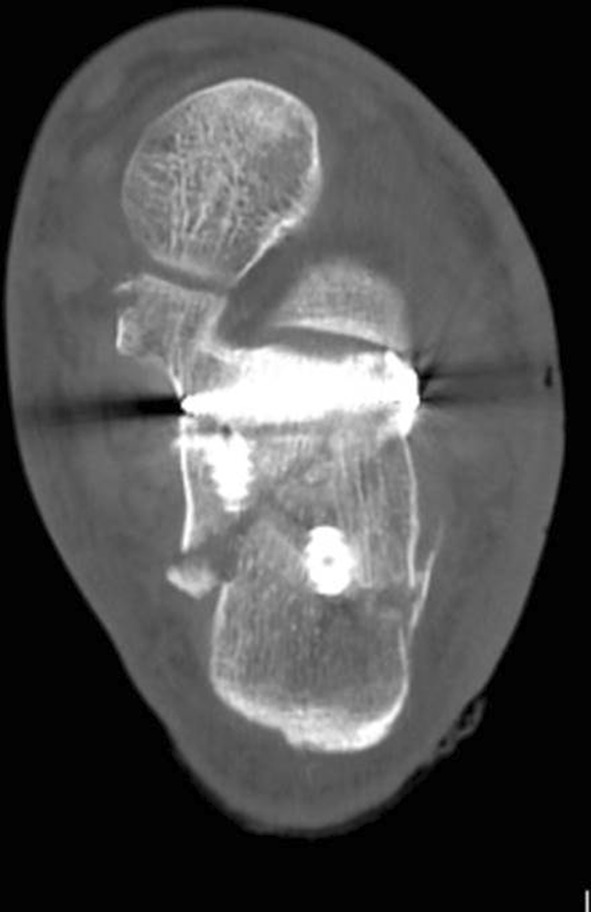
Length and lateral translation of tuberosity restored with articular reduction within 1-mm, axial view CT
